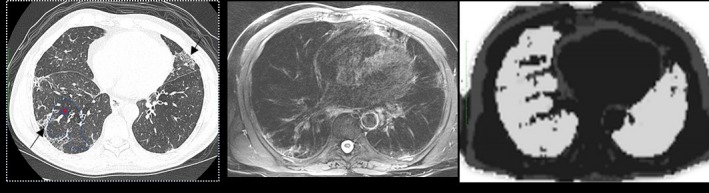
A patient with idiopathic pulmonary fibrosis. High‐resolution computed tomography (CT) [Left] shows increased attenuation areas of reticulation, traction bronchiectasis and ground glass inflammatory changes (black arrows) and low attenuation areas related to obstructive small airways disease (red circle and surroundings). The T2 Blade high‐resolution fat saturation magnetic resonance (MR) image [Middle] acquired at the same level of CT confirms most of the fibrotic changes but is unable to define low attenuation areas due to a lack of signal and diminished spatial resolution (MR imaging — 3 mm, compared with high‐resolution CT — 0.6 mm). The MR attenuation correction map is shown on the right image. [Color figure can be viewed at http://wileyonlinelibrary.com]
